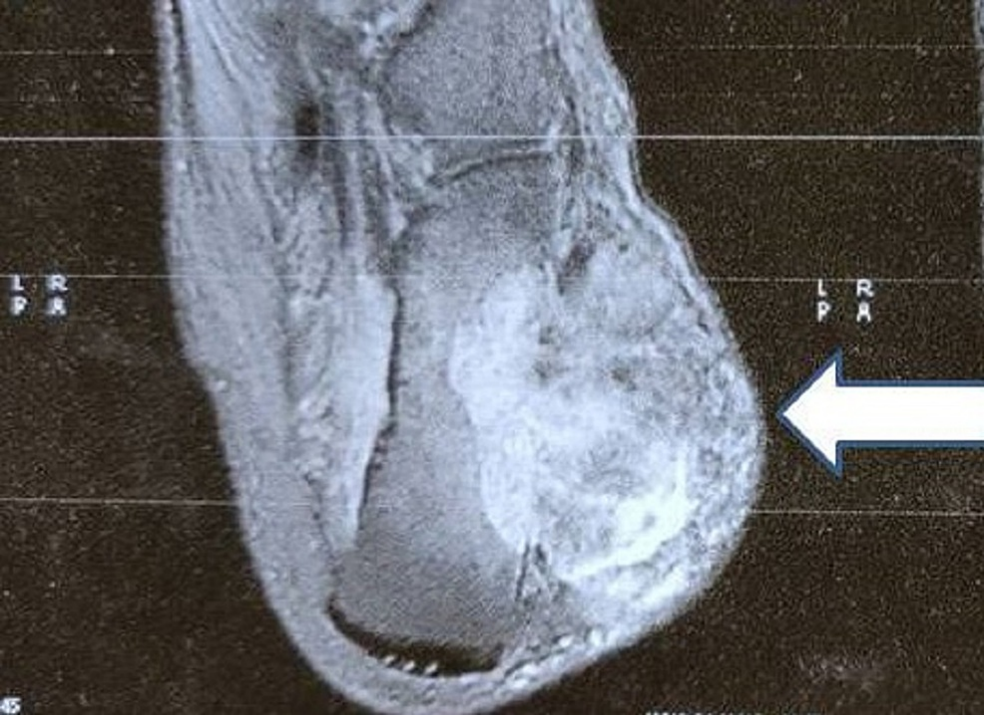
MRI showing the mass involving the calcaneum and subcutaneous tissue MRI: Magnetic Resonance Imaging
